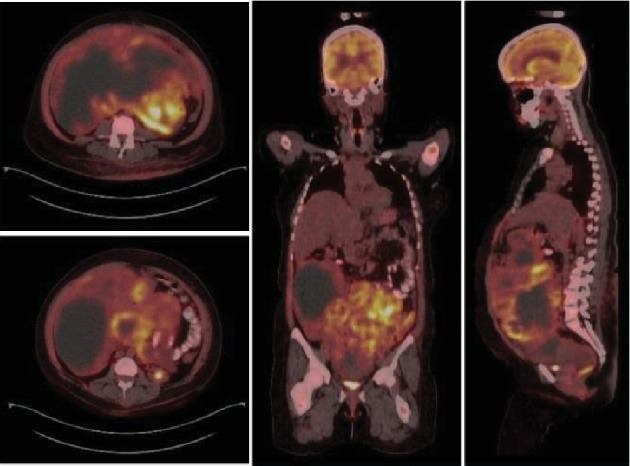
Whole-body PET CT showing multiple amalgamated masses exhibiting peripheral patchy FDG activity with low-grade FDG avid areas of peritoneal thickening/fat stranding suggesting peritoneal involvement. Low-grade FDG avid small prehepatic node measures 8 mm with diffusely activated bone marrow.
